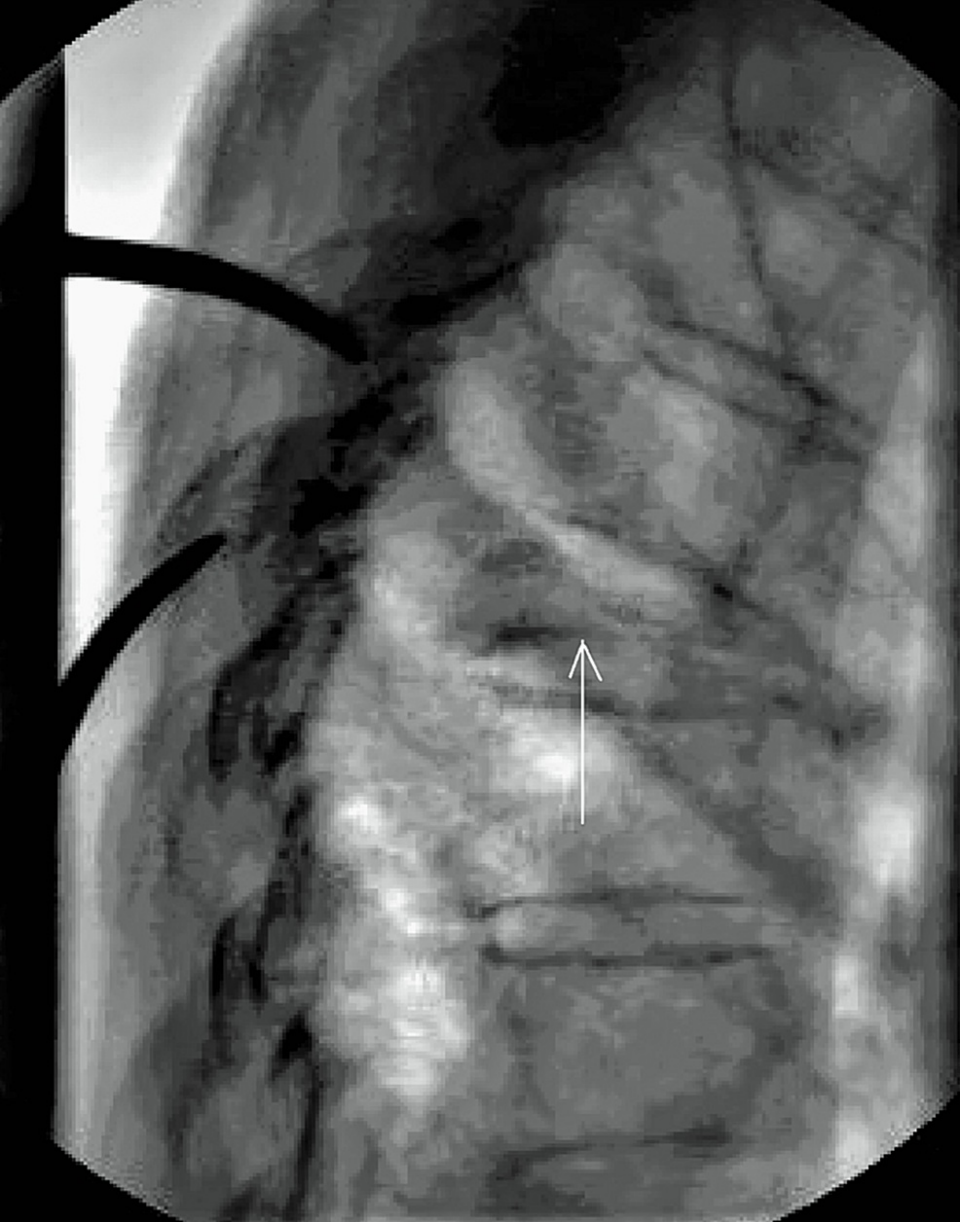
Lateral thoracic spine x-ray picture shows compression fracture (arrow) of seventh thoracic vertebral body.
